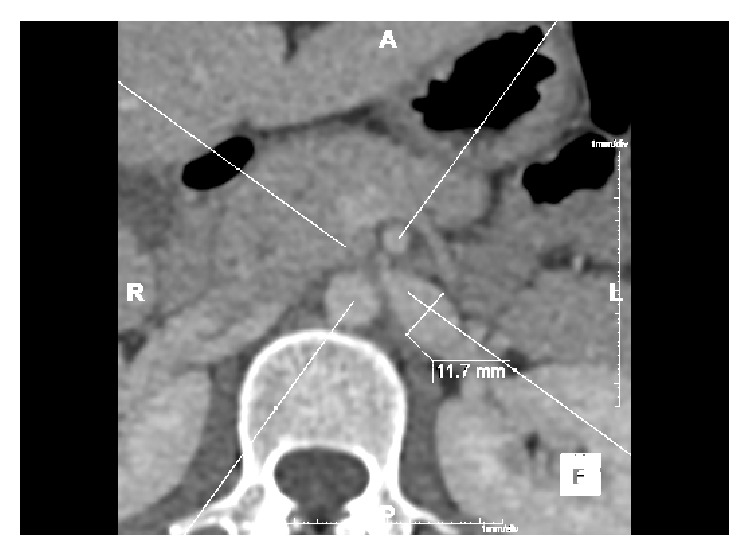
Axial contrast CT in our patient showing a grossly dilated LRV (11.7 mm) prior to impingement due to mesoaortic compression.
